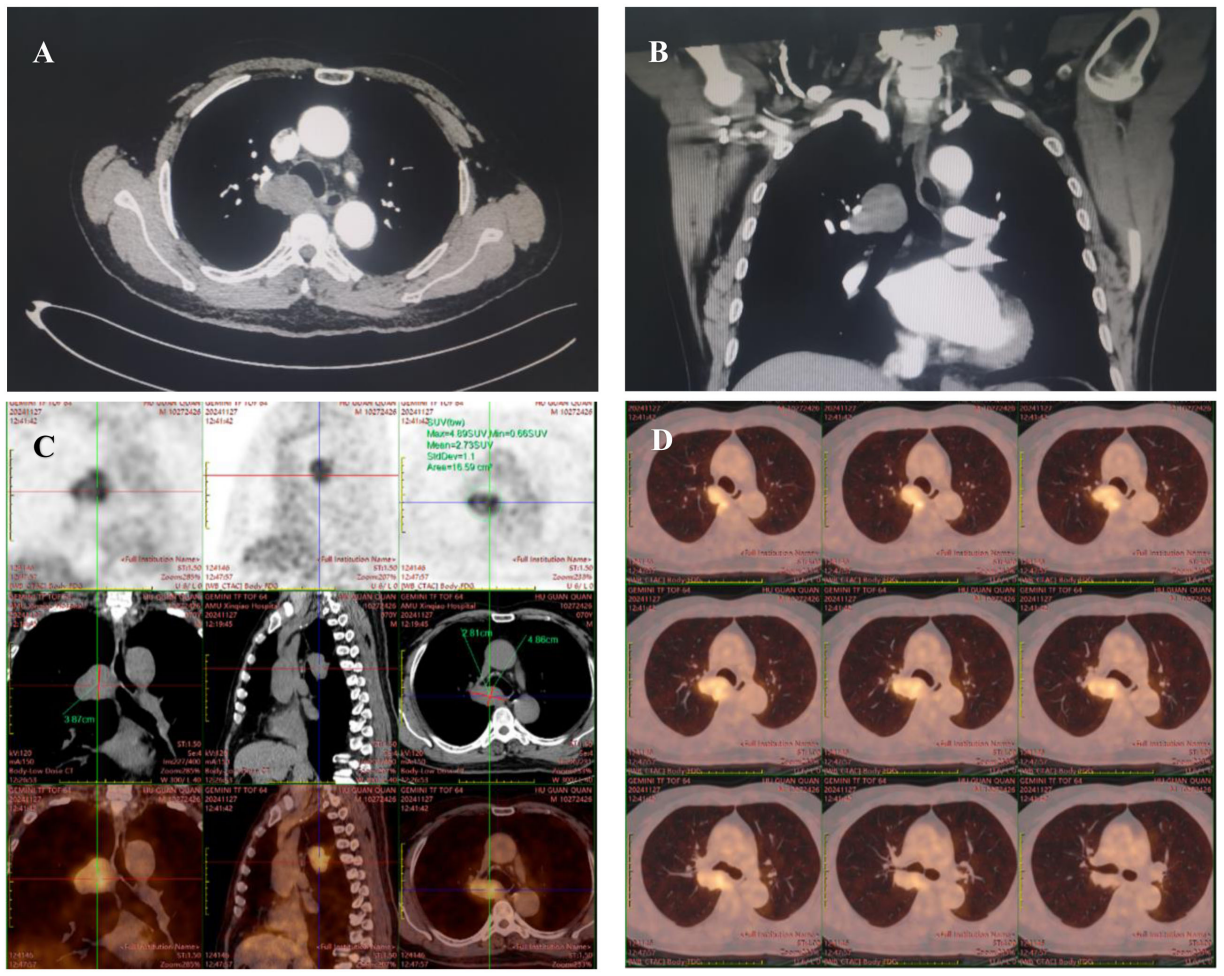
Chest computed tomography scan (A, B) reveals a 5.1 x 3.5 cm mass closely abutting the right wall of the lower tracheal segment and the right main bronchus. An 18F-FDG PET/CT scan (C, D), extending from the vertex of the skull to the upper thighs for preoperative evaluation, revealed fluorodeoxyglucose accumulation in the tumor (maximum standardized uptake value, 4.9), suggesting a suspected malignancy.
